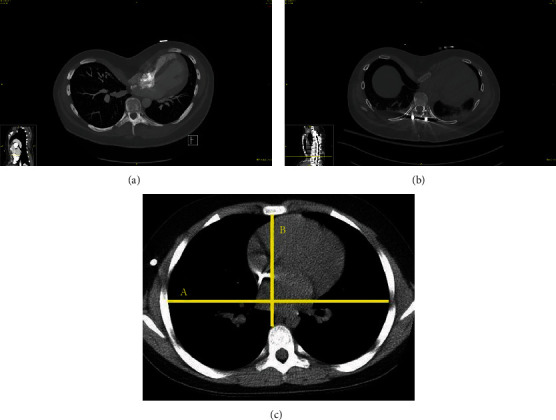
Comparison of preoperative and postoperative CT chest. Preoperative CT chest had a Haller index of 8.7. The anterior-posterior diameter minimum preoperatively was 3 cm (a). Postoperative CT chest had a Haller index of 11.3 (b). The anterior-posterior diameter minimum postoperatively was 2.3 cm. Example of a normal chest CT Haller index (c), with lines demonstrating measurements of the Haller index (ratio of A/B) [6].
